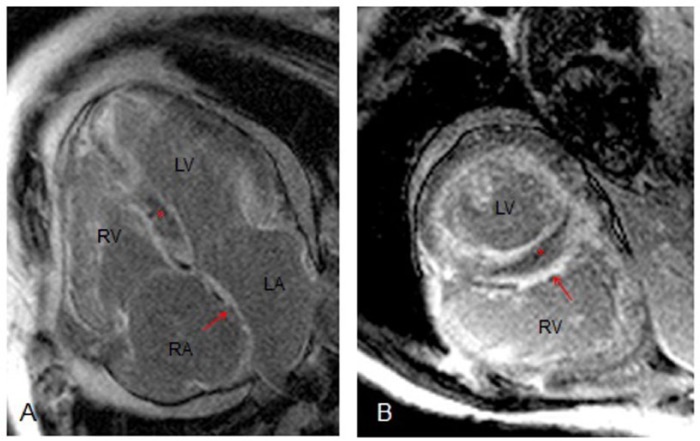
Cardiac MRI. (A) Four-chamber view demonstrating hyper enhancement (asterisk) typically found in cardiac amyloidosis and subendocardial scar (arrow). (B) Short axis view demonstrating hyperenhancement (asterisk) and subendocardial scar (arrow) pattern. Abbreviations: RA, right atrium; LA, left atrium; RV, right ventricle; LV, left ventricle.
